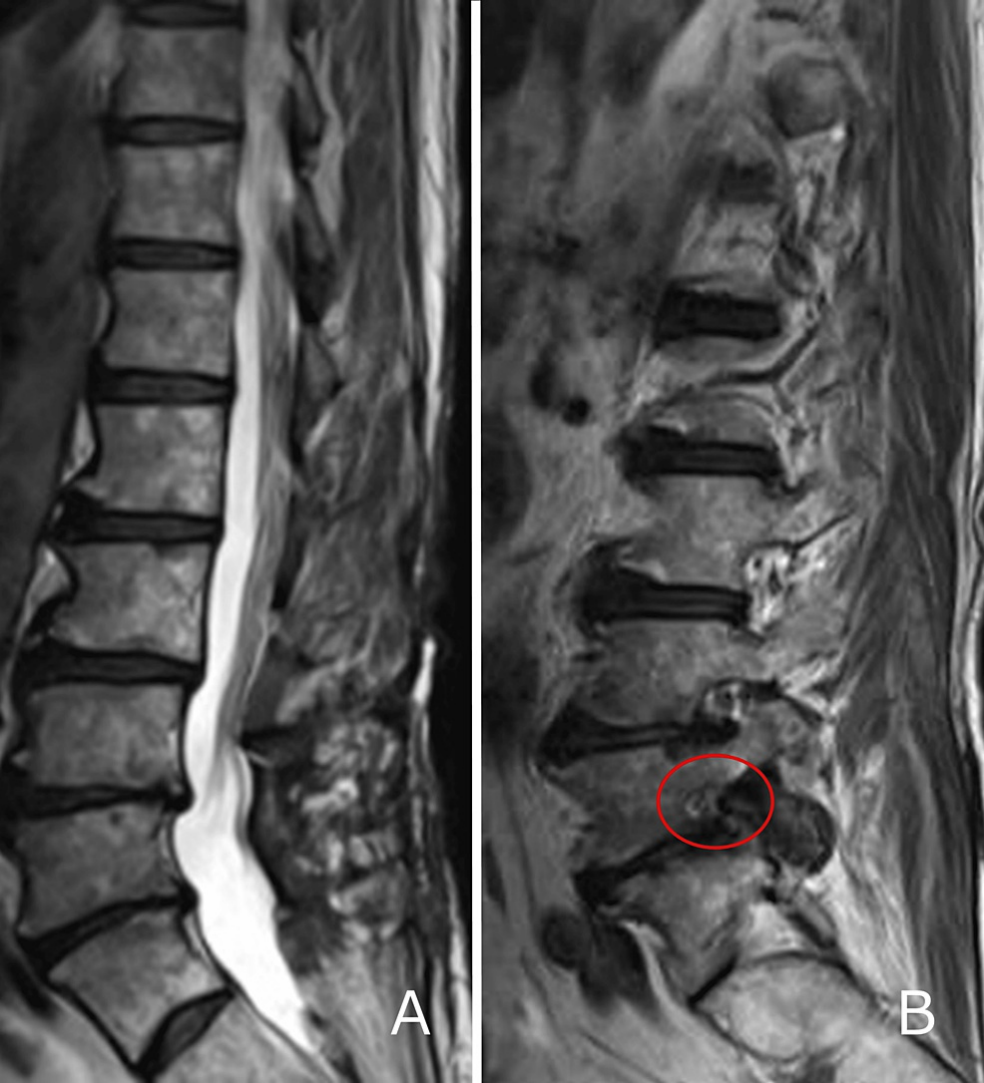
Preoperative T2-weighted magnetic resonance imaging (A) No stenosis of the spinal canal is evident. (B) Stenosis is apparent at the left L5/S1 foramen.
